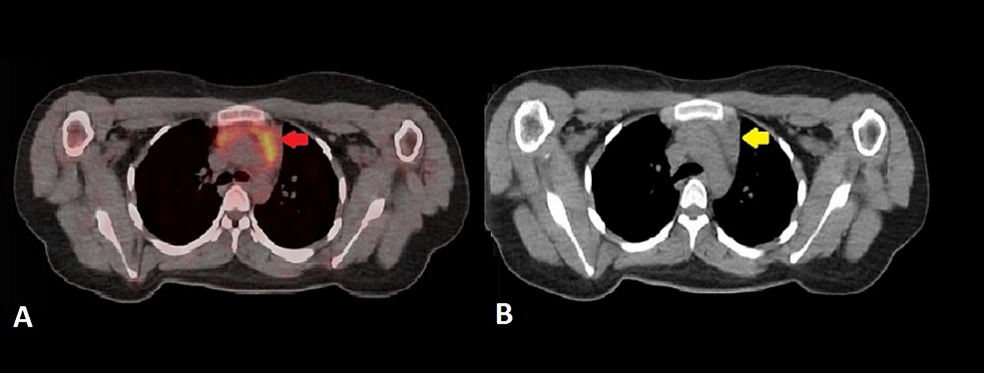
18F-FDG PET/CT axial images of upper chest and mediastinum (A) Fused image shows diffuse 18F-FDG uptake in the anterior mediastinum projecting over the thymus (B) CT image shows moderately enlarged thymus. Keeping the patient's recent history of chemotherapy, these findings represent rebound thymic hyperplasia. 18F-FDG PET/CT: Fluorine-18-Fluorodeoxyglucose Positron Emission Tomography/Computed Tomography
